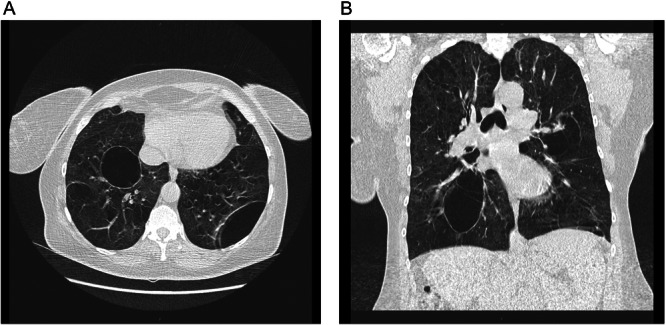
Chest computed tomography (CT) of Patient 1 (2019) in axial (A, mid‐to‐basal) and coronal (B) planes, lung window. Findings: Ground‐glass opacities with predominantly cicatricial, emphysematous, and interstitial fibrotic changes, as well as pronounced bullous alterations.
